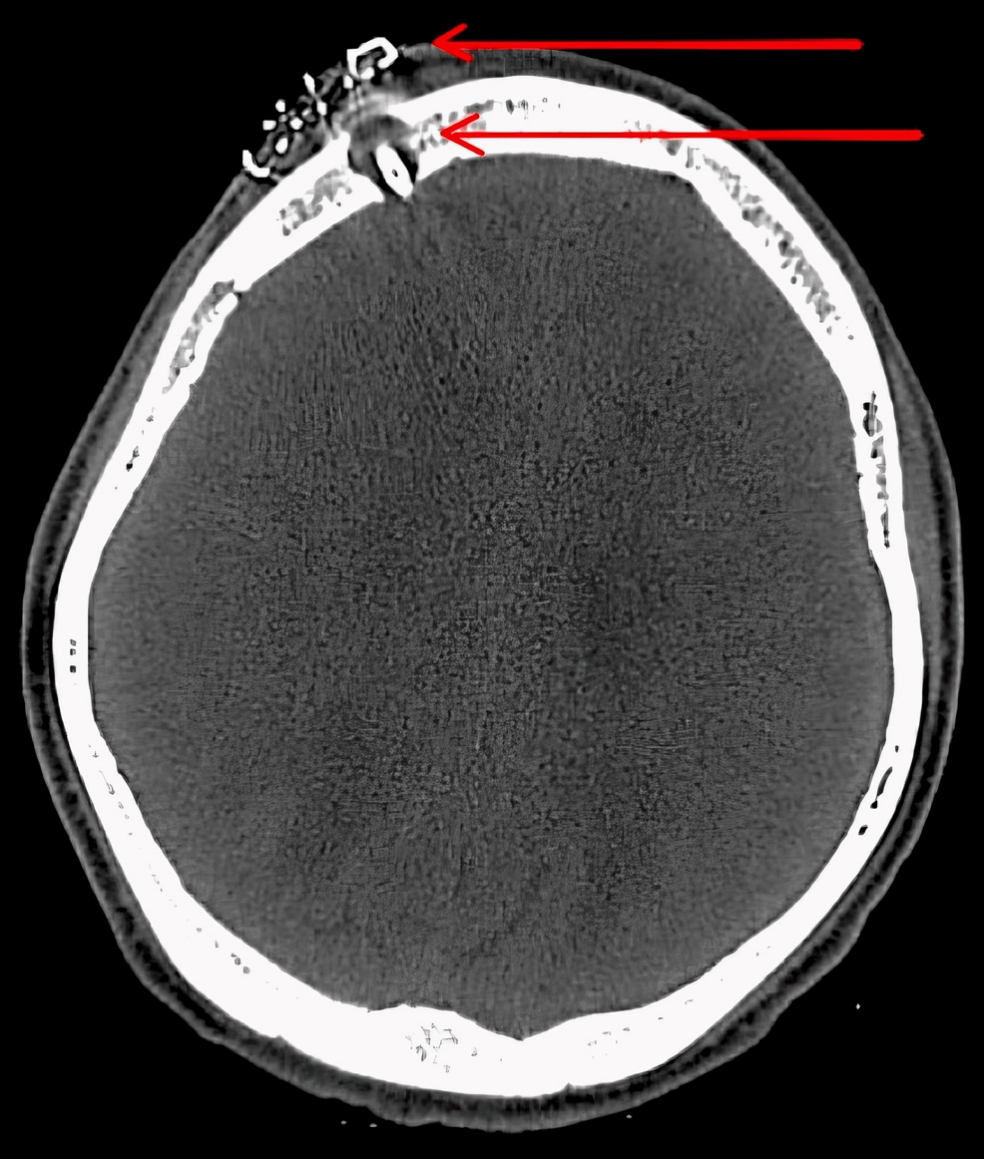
CT brain showing post-operation calvarial defect in right frontal bone showing Ommaya shunt in situ with subcutaneous post-operation sutures.
